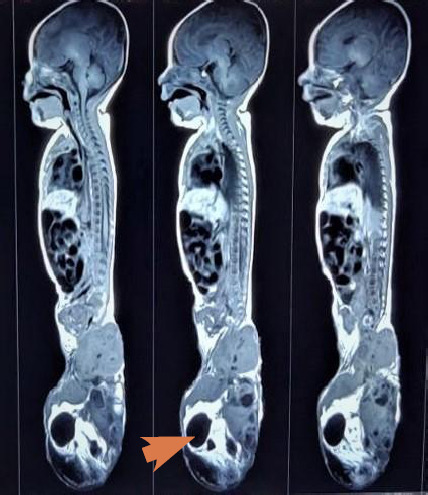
MRI of the whole neuraxis (brain and spine) showing multiloculated solid cystic
